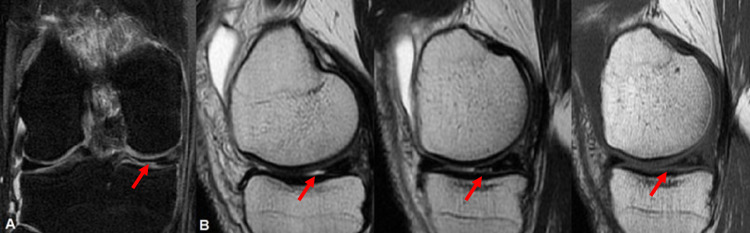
(A) MRI T2 coronal image shows partial-thickness rupture of zone 2 of the medial meniscus (red arrow). (B) MRI T1 sagittal consecutive images show the same rupture of zone 2 of the medial meniscus (red arrows).
